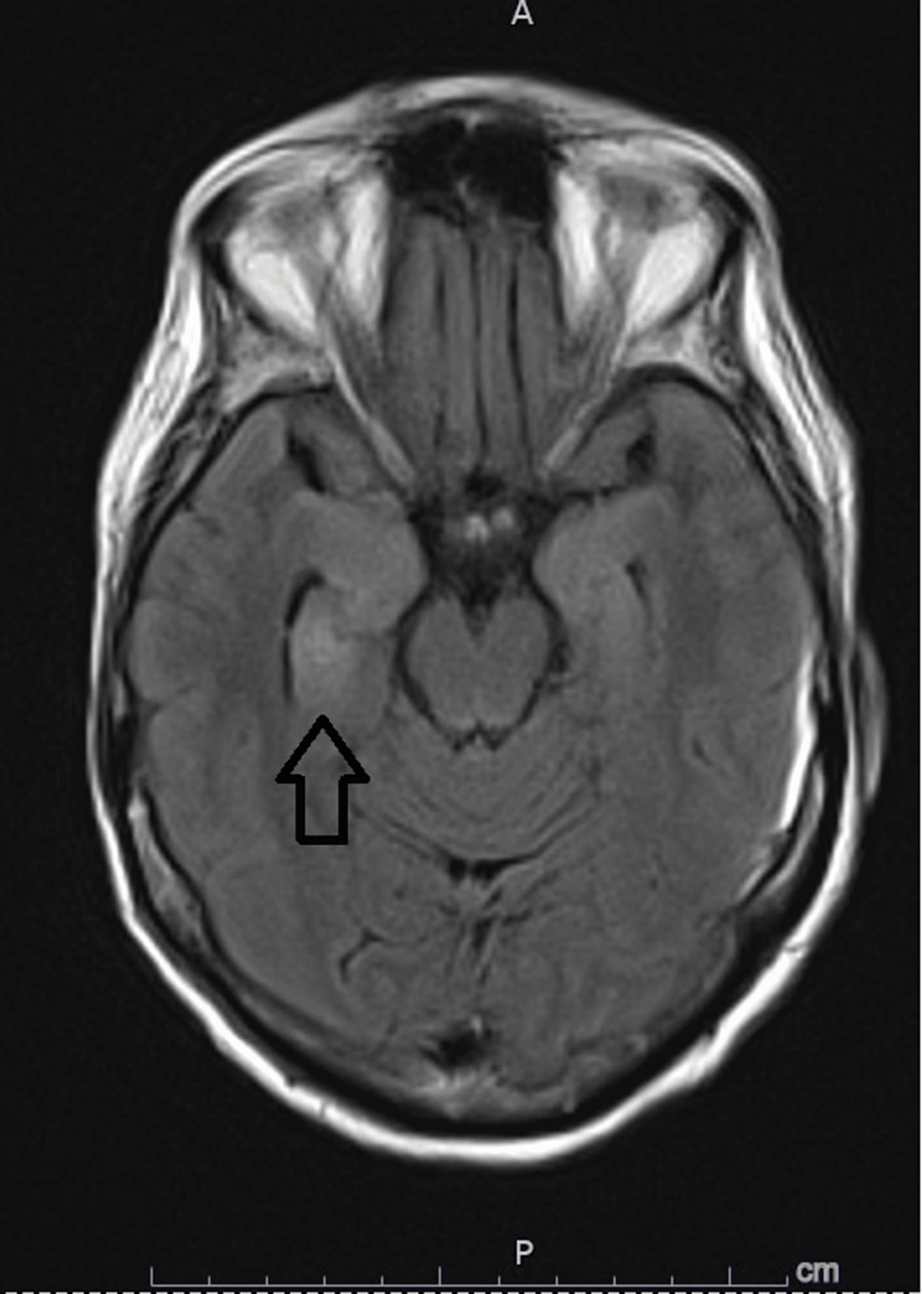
MRI Brain FLAIR MRI brain after IVIG and corticosteroid therapy showing evidence of improving right-sided temporal lobe hyperintensity (arrow).
